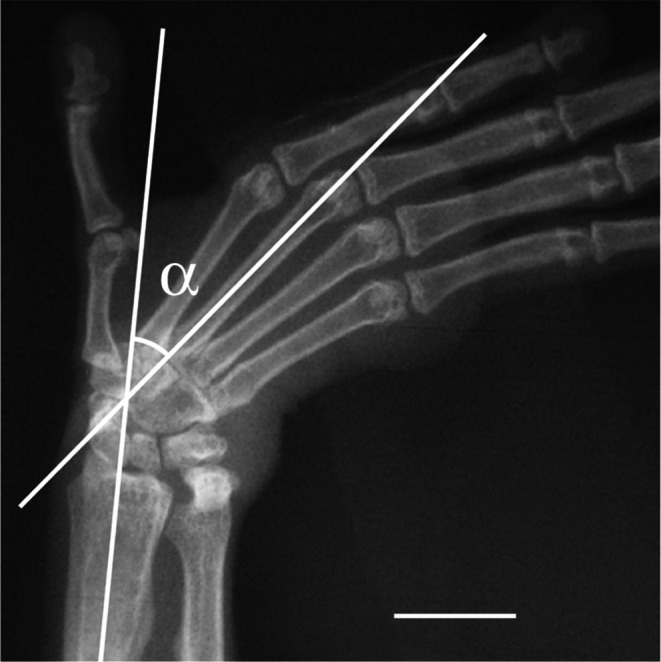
Radiograph of the hand in maximum ulnar deviation for
